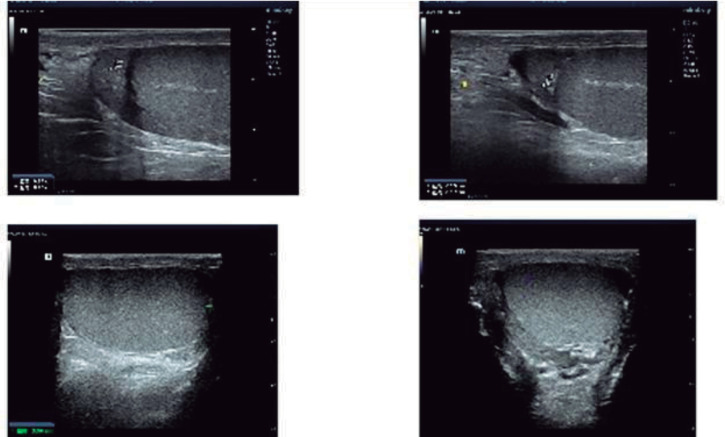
Scrotal ultrasonography. Both testicles were located in the scrotum. The right testicle measured 33.6×29.8×19.1 mm, while the left measured 36.6×25.1×19.8 mm. Each head of the epididymis contained a cyst (1.5×1.2 mm on the right side, 1.4×1.3 mm on the left side).
